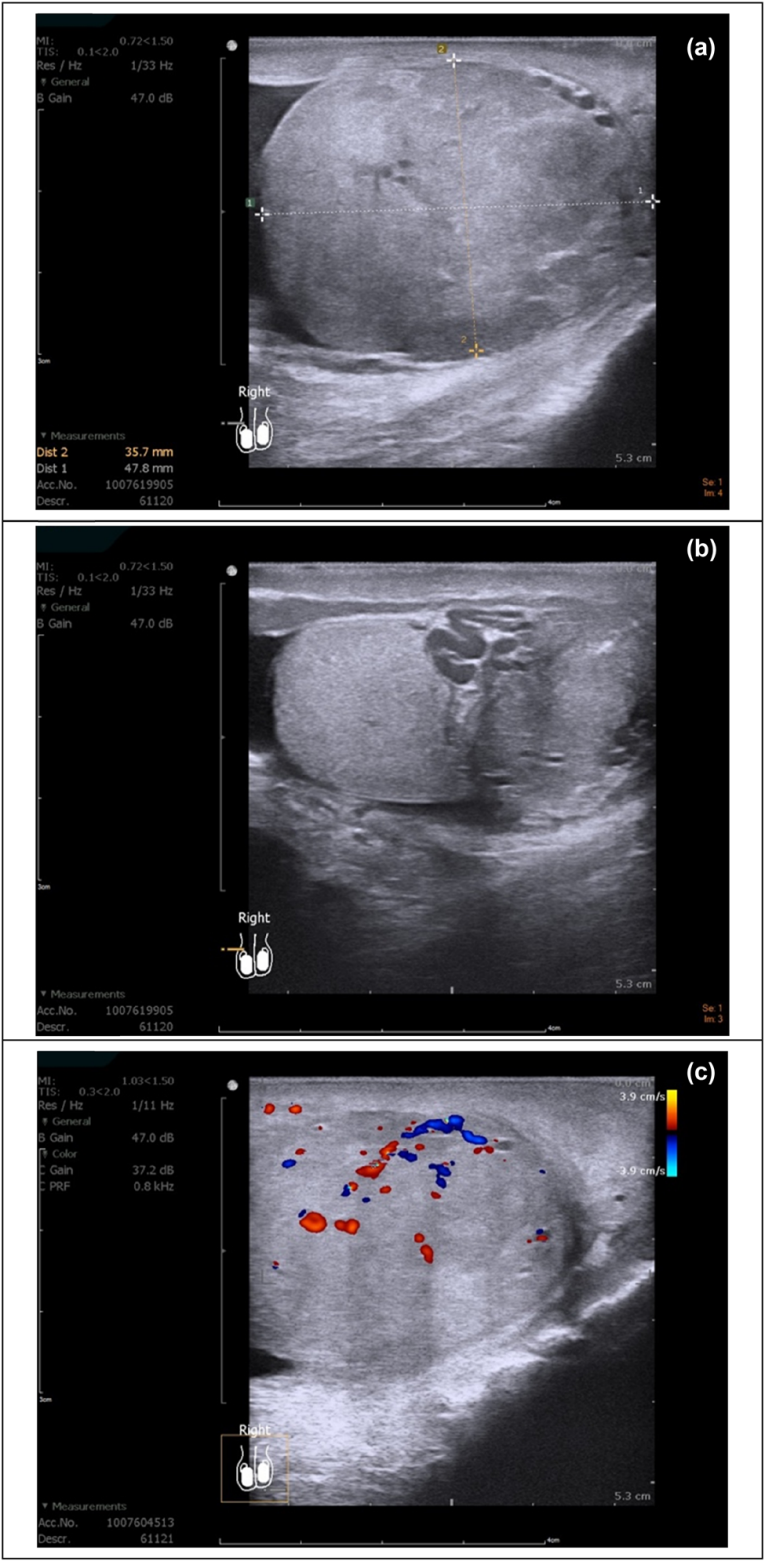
Ultrasound sonography: (a) a well-capsulated heteroechoic round mass over the tail of epididymis, 4.7 × 3.5 cm in size, and (b) abundant tortuous vessels adjacent to the paratesticular tumor. (c) The tumor had a rich blood supply on color Doppler sonography.
